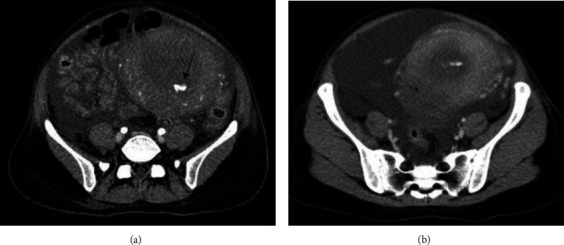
(a) Contrast-enhanced computed tomography images at the time of massive postpartum hemorrhage: extravasation from the uterine cavity (→). (b) massive ascites.
